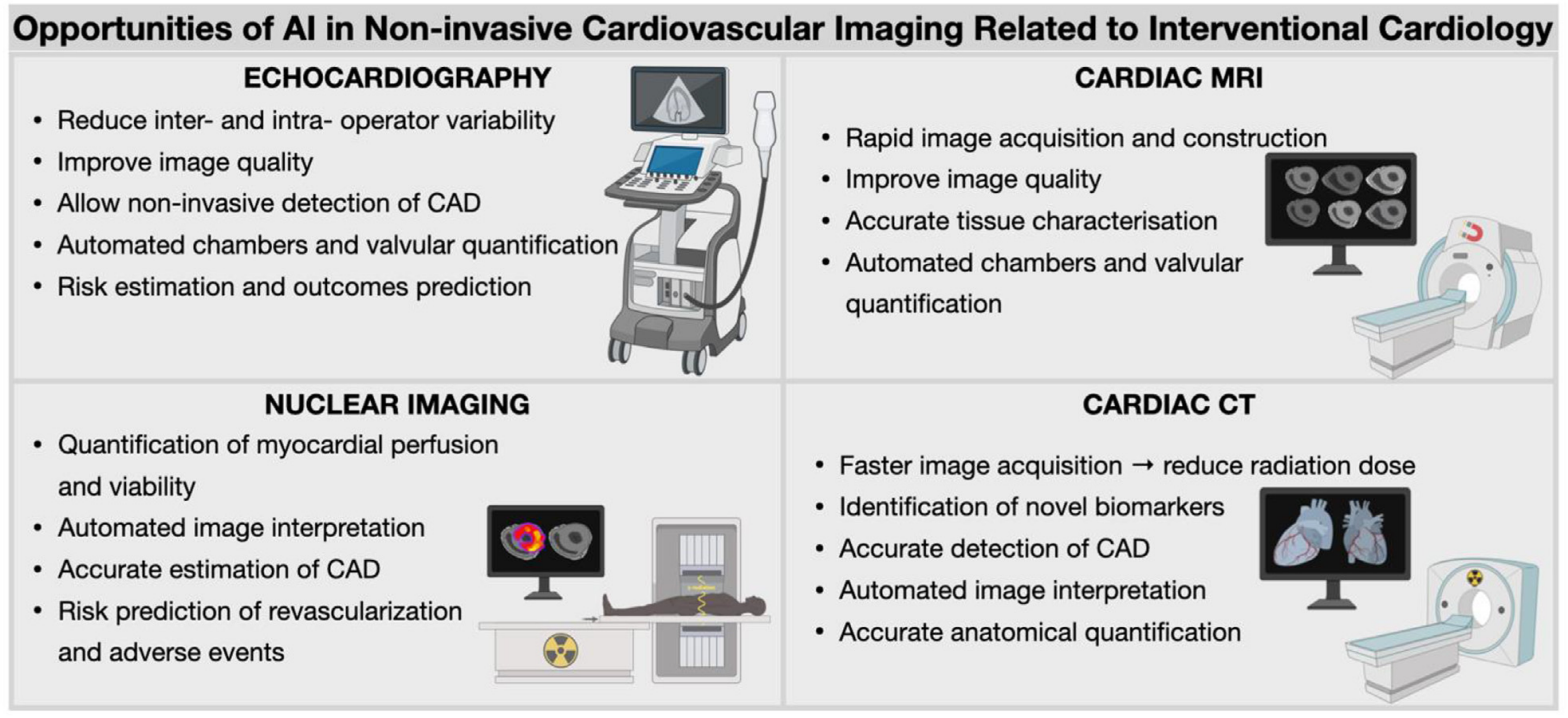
Potential role of artificial intelligence (AI) in noninvasive cardiac imaging related to interventional cardiology. CAD, coronary artery disease; CT, computed tomography; MRI, magnetic resonance imaging.
